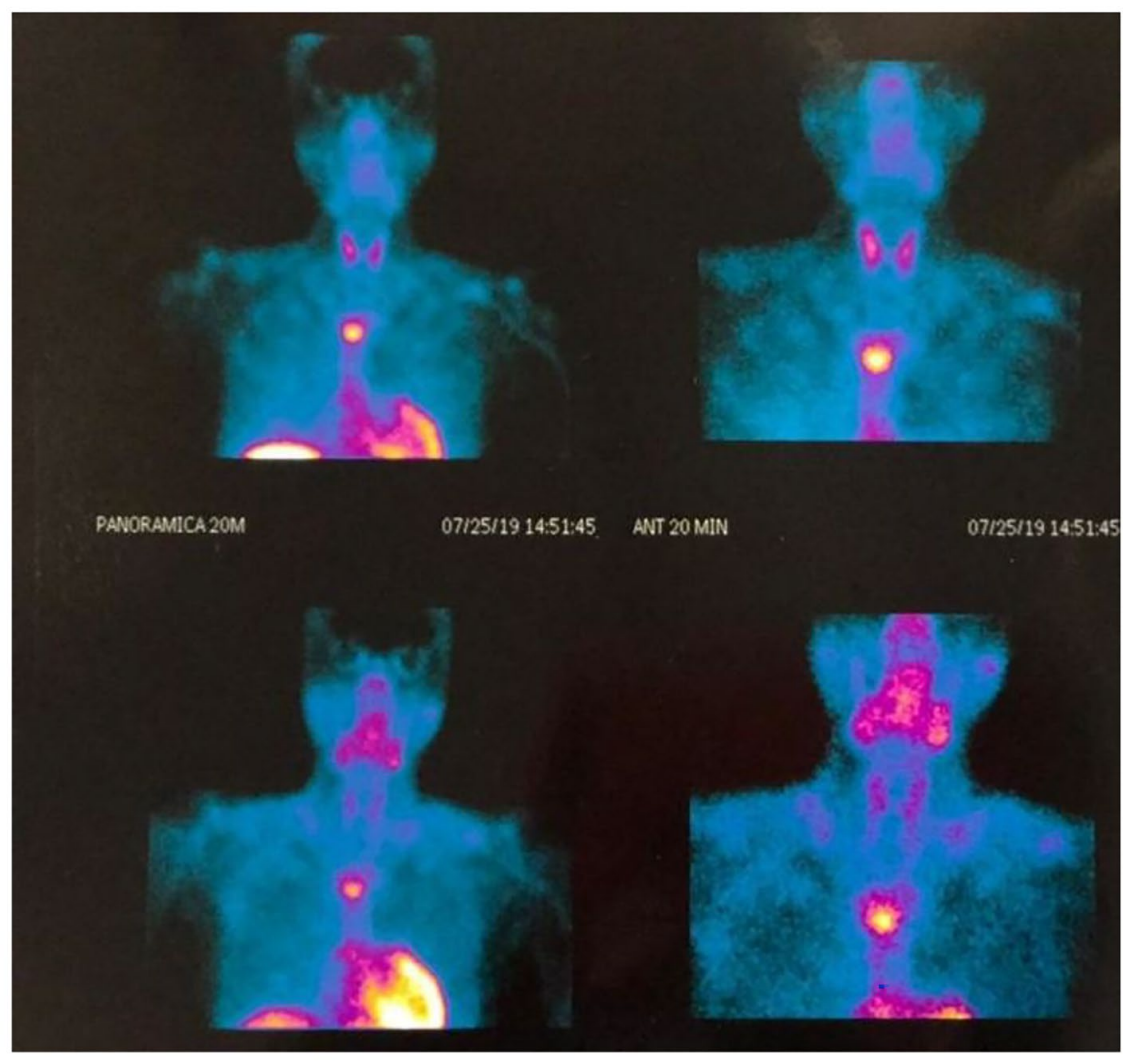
Results of a 99mTc Sestamibi SPECT scan in a patient with a MEPA in the medium mediastinum
